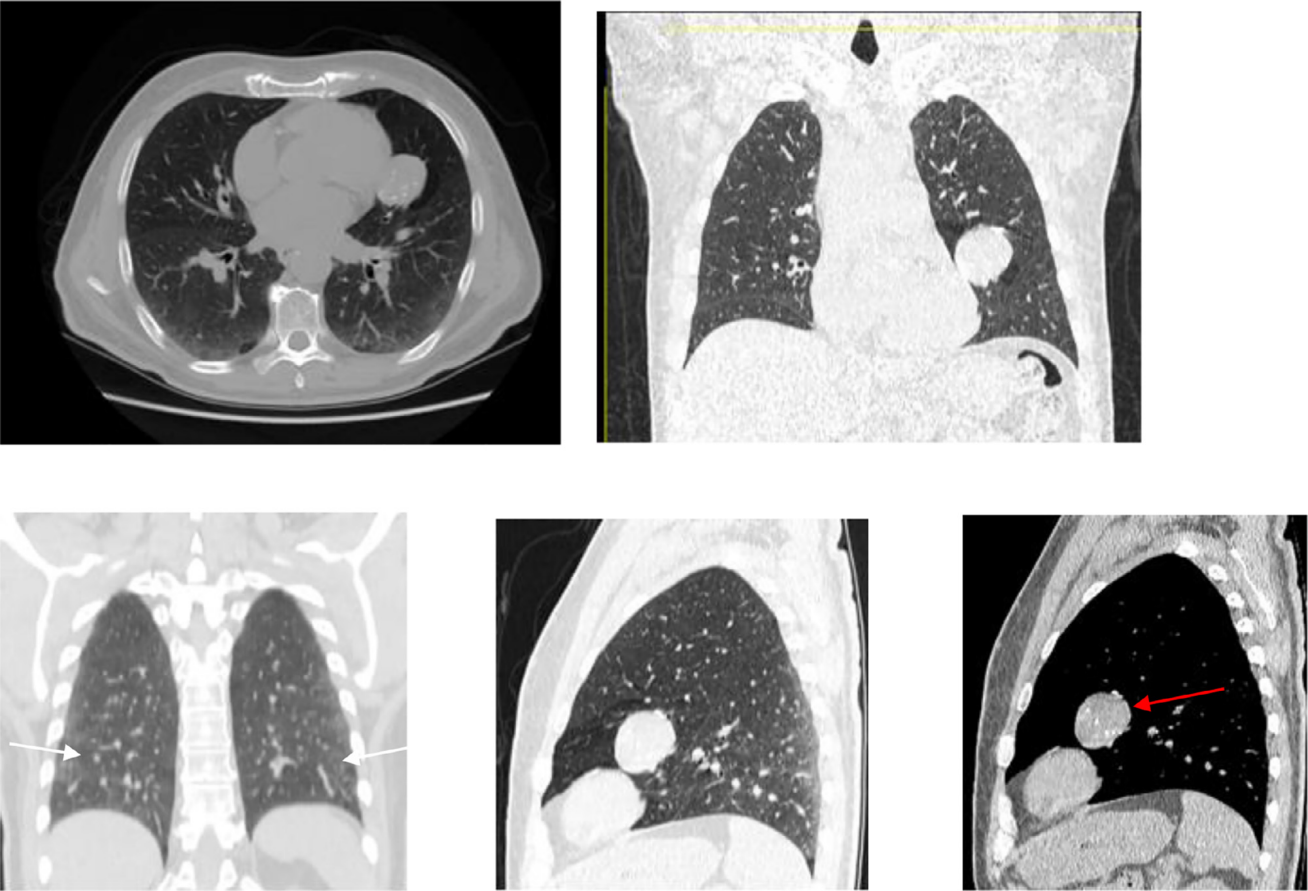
(a–e): CT showed in the basal regions of both lungs the presence of initial areas with “ground glass” pattern, with interstitial thickenings, as initial Covid-19 bilateral pneumonia (white arrows). CT also demonstrated a solitary, large (4 × 4 cm) mass lesion, in the left lung, with numerous and minute intralesional calcifications (“popcorn” calcifications; red arrow). (Color version of figure is available online.)
